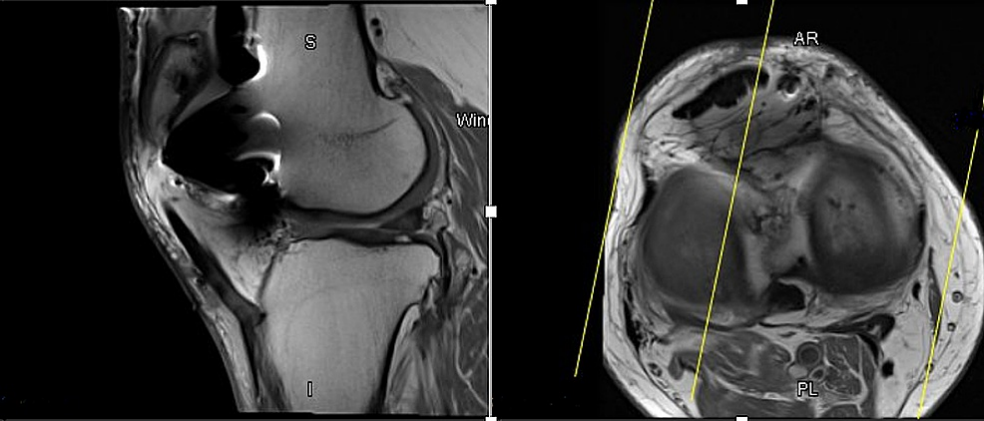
Magnetic resonance images of the right knee displaying lateral meniscus tear. The left image is a sagittal proton density sequence slice of the right knee in the lateral compartment displaying a lateral meniscal tear and artefact from the patellofemoral implant. The right image is a proton density fat suppressed sequence axial slice at the level of the tibial plateau displaying a lateral meniscal tear.
